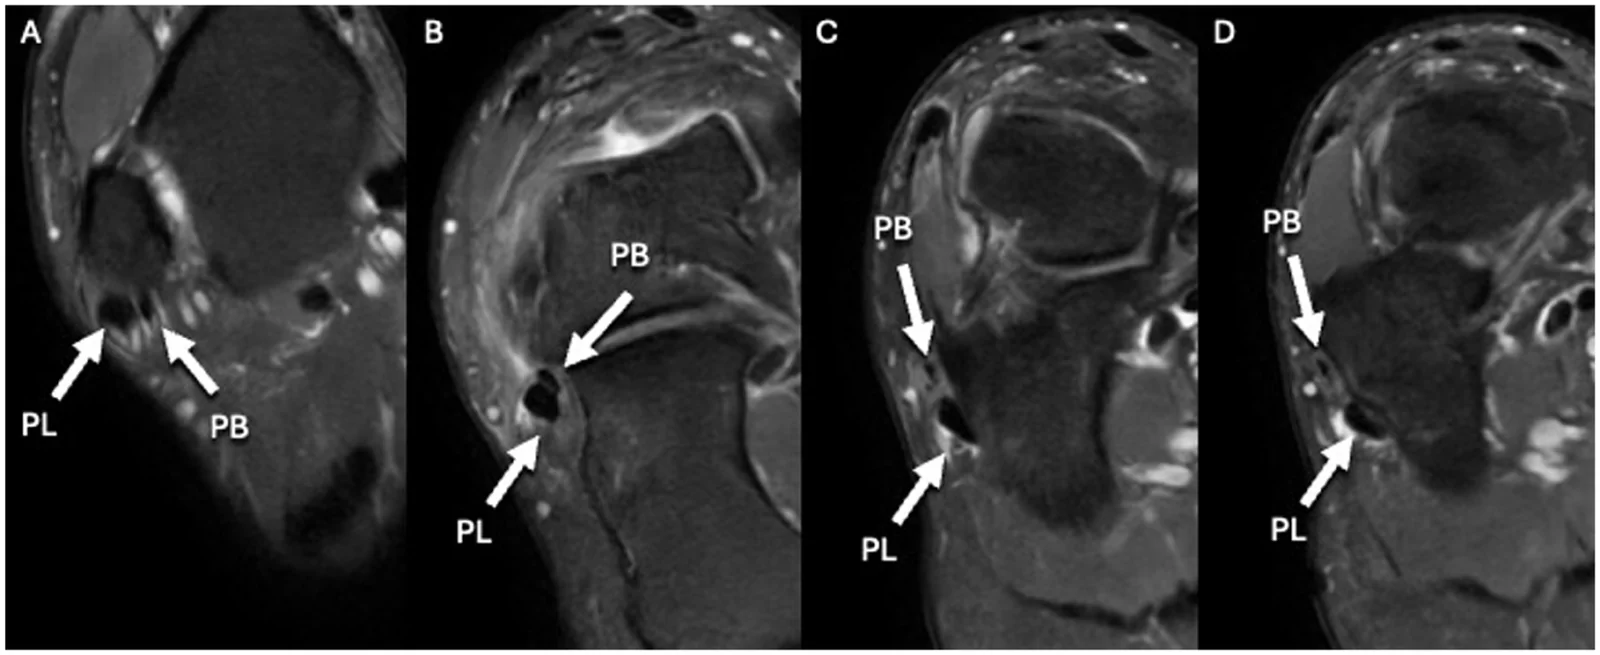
Axial oblique proton density fat-suppressed MRI images of the right ankle (A–D, proximal to distal). The peroneus brevis tendon courses “normally” without a bifid configuration but is diminutive distally, becoming difficult to trace near the calcaneocuboid joint. PB, peroneus brevis; PL, peroneus longus.
